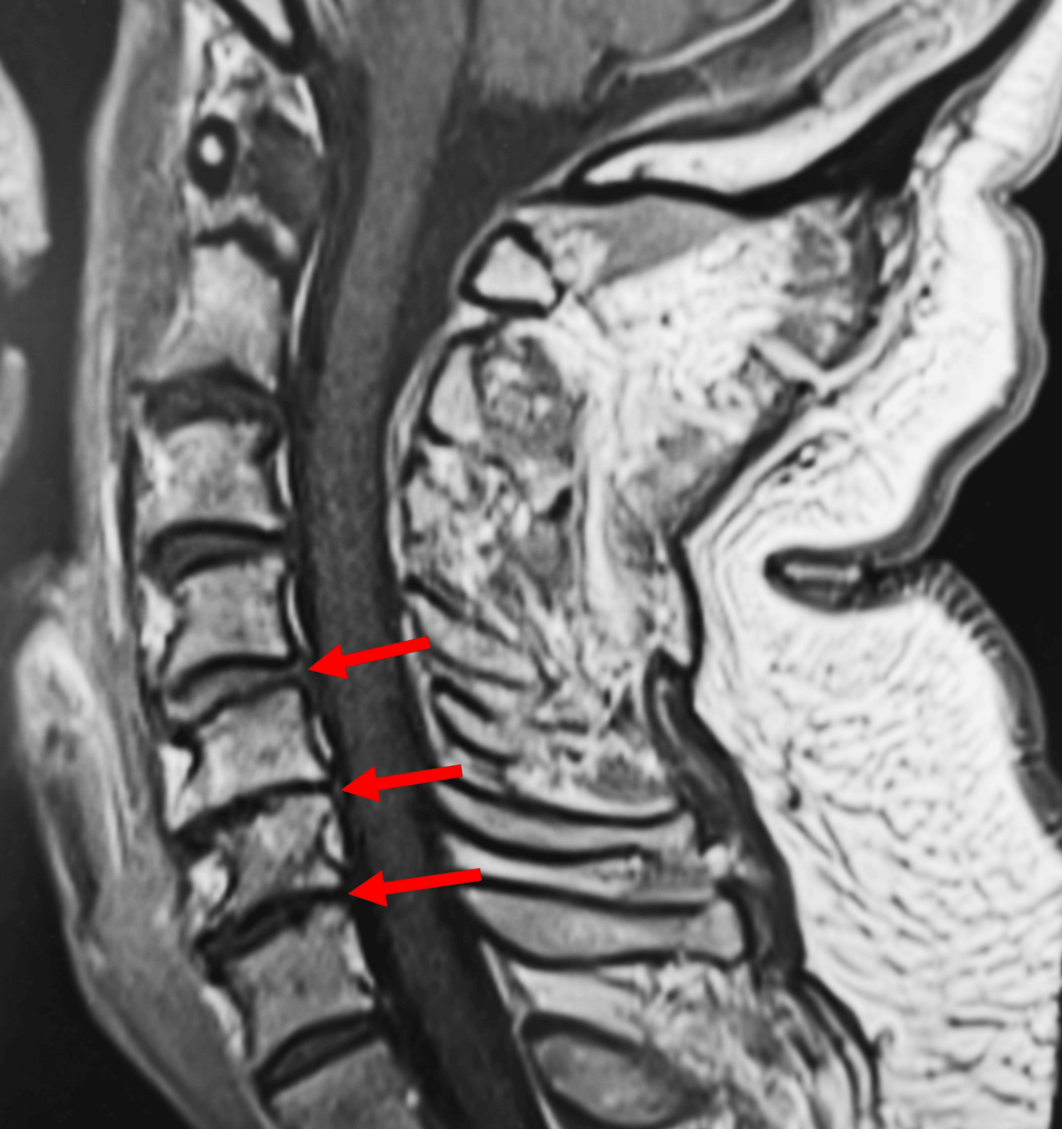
Cervical spine MRI on day two of hospital admission showed degenerative disc disease A cervical MRI on day two of hospital admission showed degenerative disc disease at C3-C6 (red arrows), but normal cord signal intensity.
